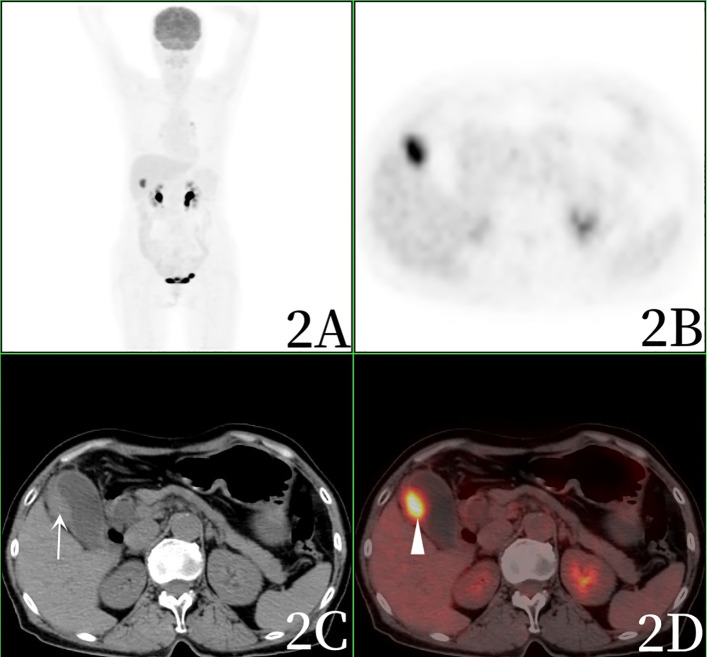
Female, 69 years old.1h after injection of 18F-FDG into PET/CT, 2A (whole body MIP), 2B (axial PET), 2C (axial CT), 2D (axial fusion) showed slightly increased gallbladder volume, uneven thickening of gallbladder bottom, eccentric and limited thickening of gallbladder body wall (arrow), and nodular soft tissue density shadow with a size of about 13×19mm. The hepatobiliary interface was clear, radioactivity uptake increased, and SUVmax was 10.2 (arrow).
